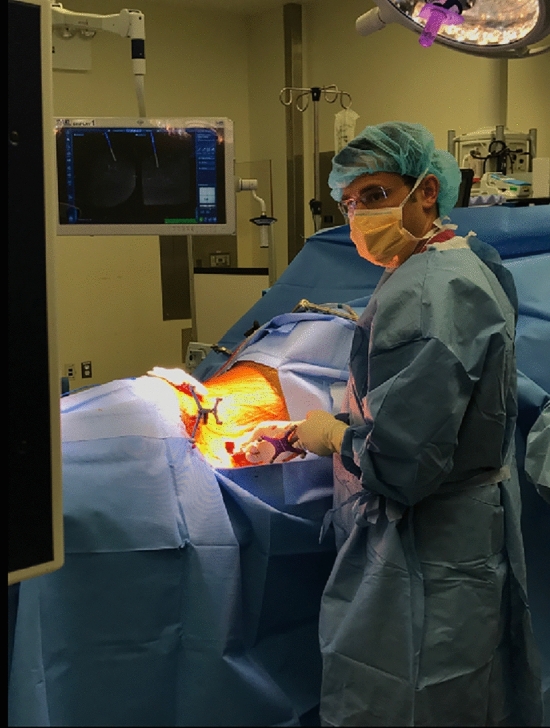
Percutaneous pedicle screw placement with the patient in lateral decubitus position performed using the CT based 3-dimensional respresentation of the patient’s spine in the navigations system
